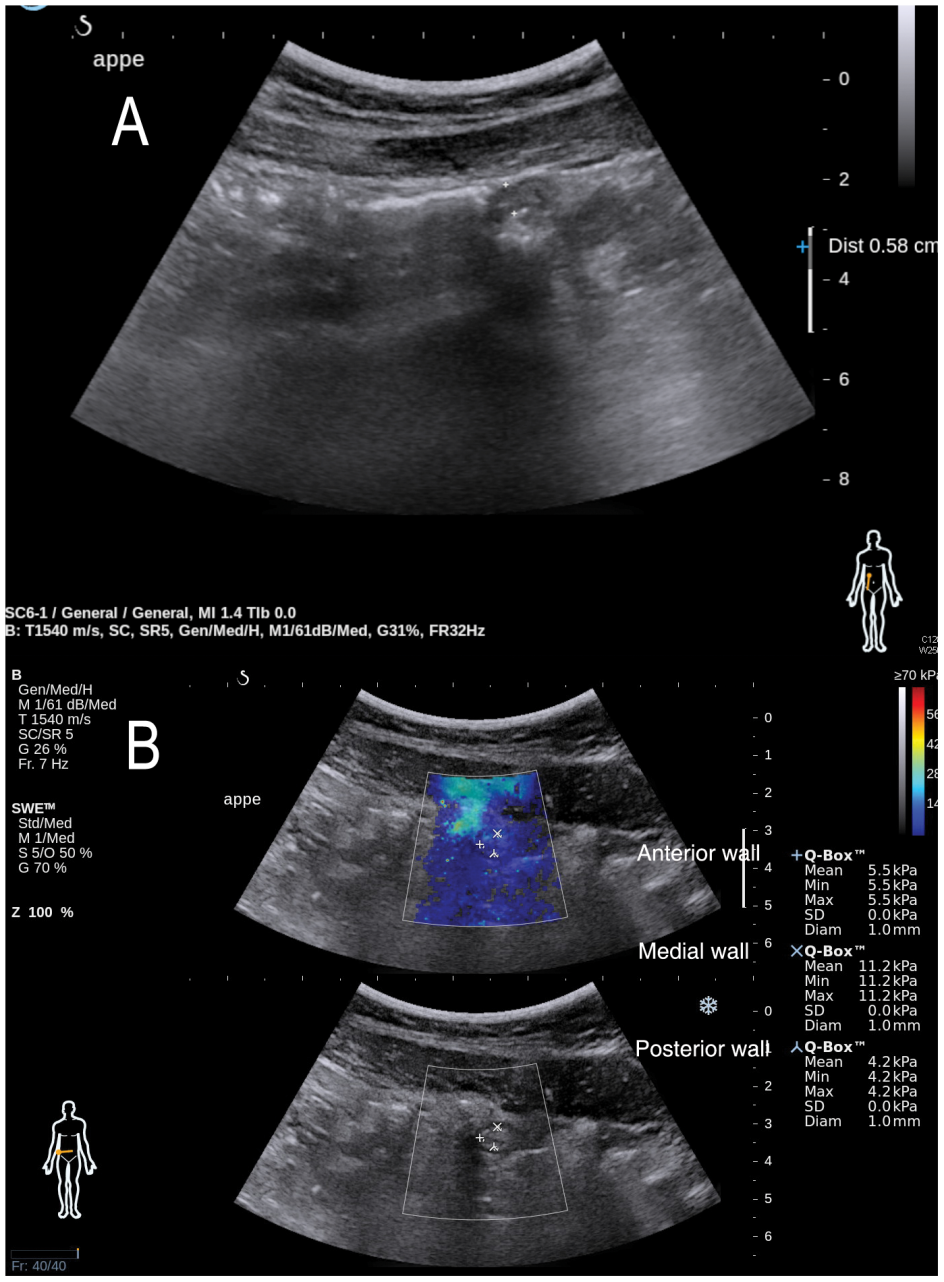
Gray-scale ultrasonography and shear wave elastography in a 24-year-old male patient with no appendicitis. A. Gray-scale ultrasonography was 5.8 mm in diameter. No echogenicity of periappendiceal fat or appendiceal wall thickening were noted. B. Elastic modulus scales (mean Q-Box) by shear wave elastography were 5.5, 11.2, and 4.2 kilopascal (kPa) in the anterior, medial, and posterior wall of the appendix, respectively. The highest elastic modulus scale (11.2 kPa) was selected for analysis. The modified Alavarado score in this patient was 5, and computed tomography showed a smaller diameter (<6 mm), no enhancement of periappendiceal fat, and the absence of appendiceal wall thickening. This patient was diagnosed with terminal ileitis and recovered without surgical intervention.
